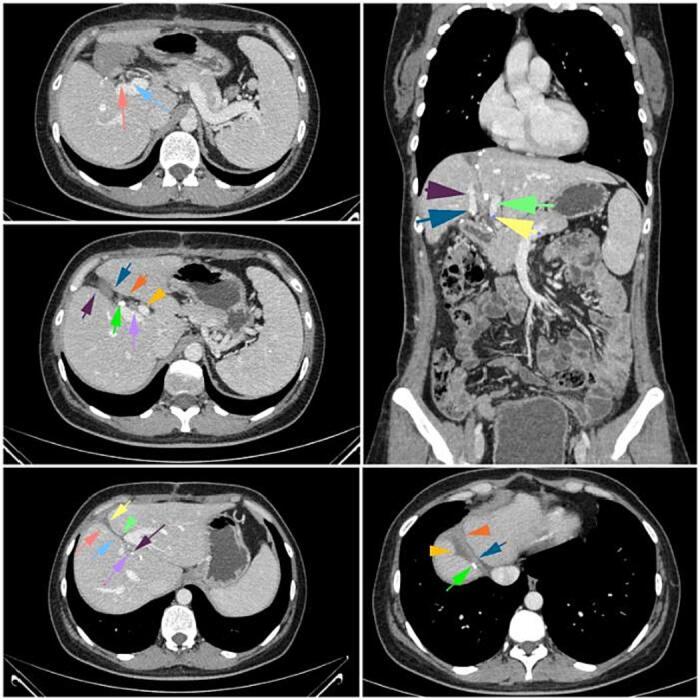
CT imaging at the 18th month after the surgery.
